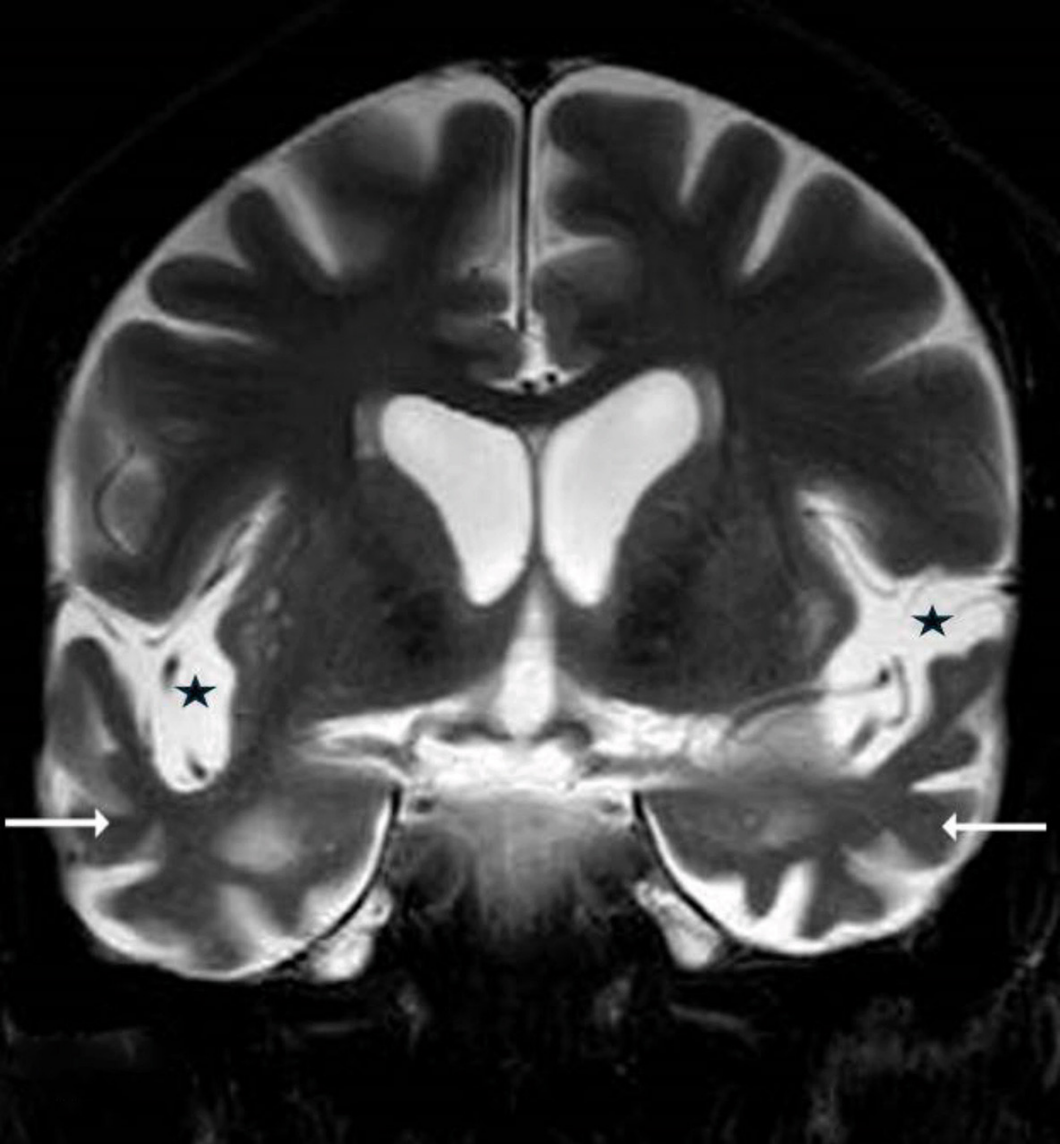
Coronal T2W sequence of the brain showing widened sylvian fissures on both sides (black asterisks) with atrophy of both temporal lobes (long white arrows). T2W: T2-weighted.
